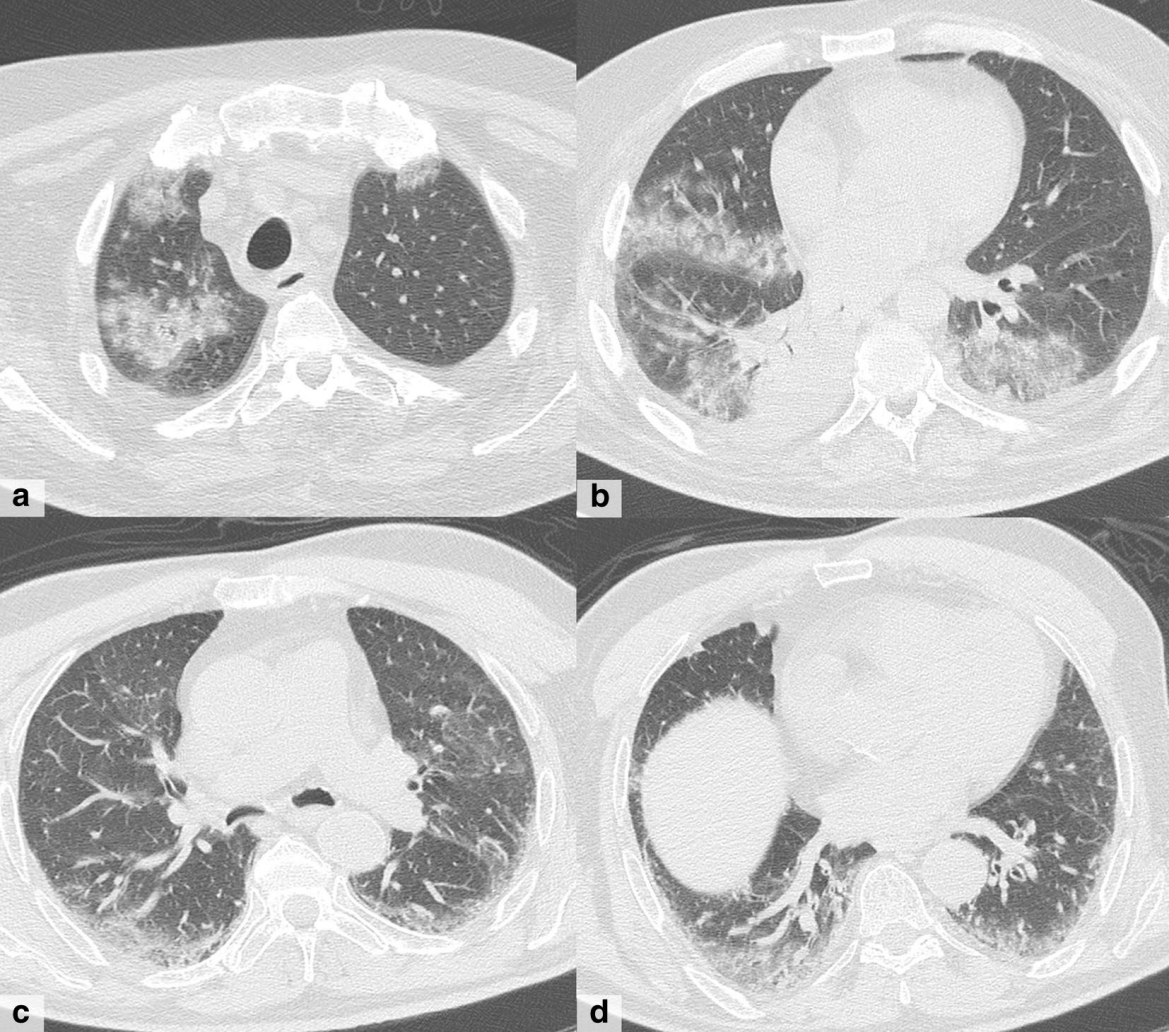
Two cases of discrepancies between CT findings and RT-PCR results. a–b A 57-year-old man with diabetes and hypertension, admitted in critical condition for serious dyspnea and stupor. CT shows bilateral and mostly peripheral multifocal confluent areas of ground-glass opacity with a wide area of consolidation in the lower right lung (b). RT-PCR result was negative, and the final diagnosis was Klebsiella pneumoniae infection. c–d A 83-year-old man with cardiomyopathy and diabetes who presented with fever in the last 3 days and history of COVID-19 exposure. CT shows thin semilunar symmetric areas of peripheral subpleural increased density, bronchial wall thickness, signs of vascular congestion and cardiomegaly; these signs were interpreted as congested interstitial spaces and poorly aerated zones secondary to bronchitis and heart dysfunction. Instead, RT-PCR result was positive
